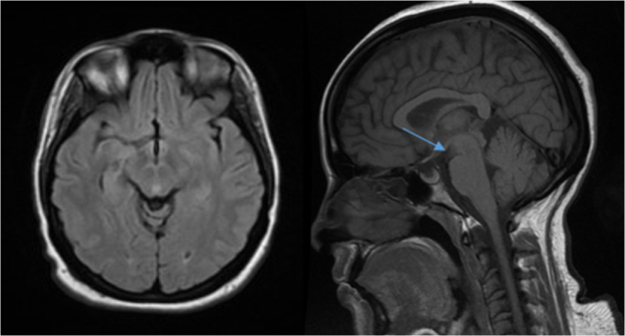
Follow-up MRI showing resolution of FLAIR hyperintensities and mammillary body atrophy.
